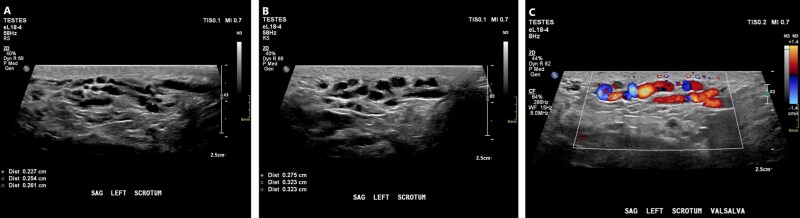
Left sided varicocele. (A) Within the left scrotum, there are enlarged tubular structures measuring up to 2.6 mm in diameter. (B) With Valsalva maneuver, the tubular structures dilate up to 3.2 mm. (C) There is flow on Doppler interrogation confirming that these tubular structures represent veins of the pampiniform plexus.
